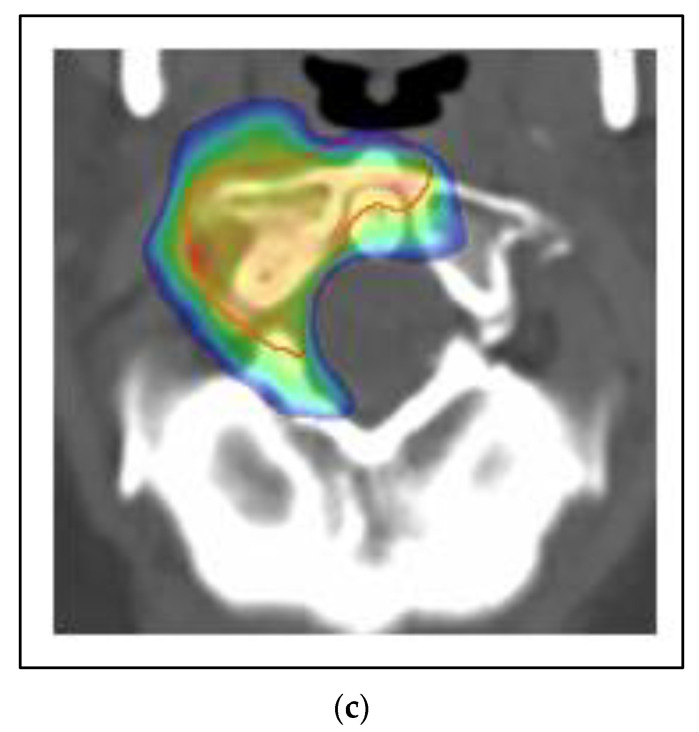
Case 4: Oligoprogression in the C1 vertebra. A 45-year-old woman presented with oligoprogression at C1 on systemic therapy for metachronous bone metastases. Representative images of the C1 metastasis on PET/CT (a) and MRI (b). (c) A colorwash image superimposed on a radiation planning CT showing the dose from SBRT to the C1 vertebral metastasis 24 Gy in 2 fractions with photon IMRT.
